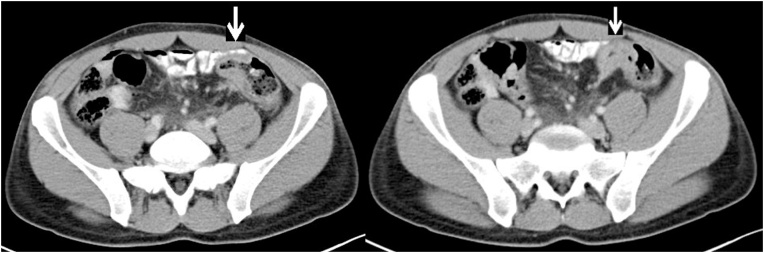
Abdominal CT revealed stenosis at the transition of the descending to sigmoid colon.
